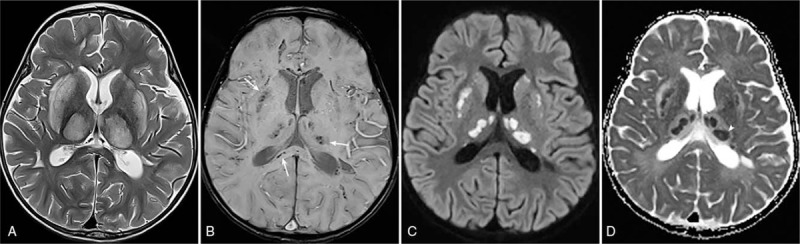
Recurrent episode of ANE in Case 1 following febrile respiratory illness. (a) Axial T2-weighted image shows symmetrical and bilateral T2 hyperintensity involving both the thalami and basal ganglia. The brainstem (images are not shown) was also involved. (b) SWI shows petechial hemorrhages (arrows). (c) and (d) DWI shows multifocal areas of diffusion restriction in both the basal ganglia and thalami; the ADC map shows the tricolor pattern (arrow heads). ADC = apparent diffusion coefficient, ANE = acute necrotizing encephalopathy, DWI = diffusion-weighted image, SWI = susceptibility-weighted image.
